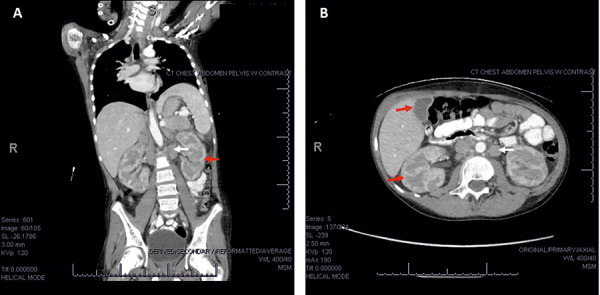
Computed tomography scan of the chest, abdomen, and pelvis from the patient’s second admission. Axial (A) and coronal (B) images show enhancing tumors primarily invading the kidneys, adrenal glands, and liver (red arrows). Retroperitoneal adenopathy is also noted (white arrows).
